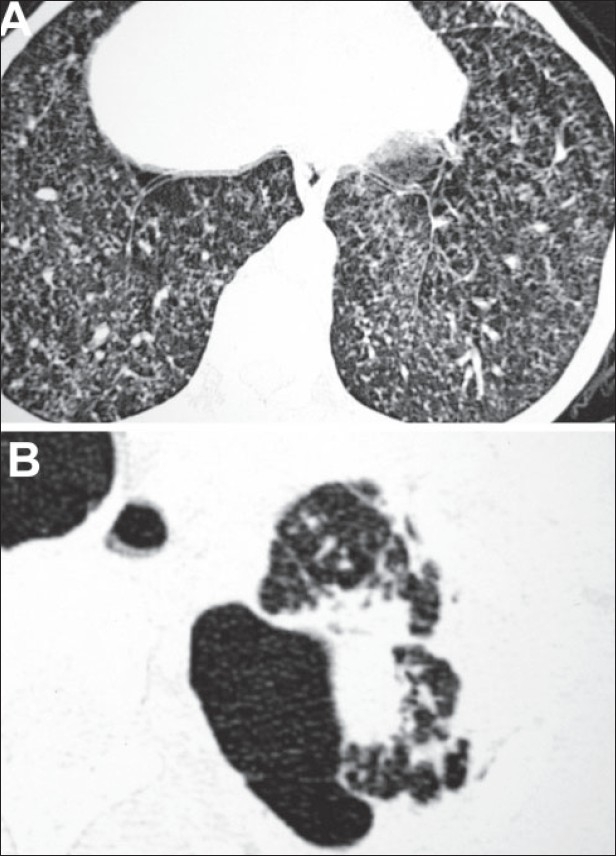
A. Computed tomogram revealing miliary opacities over bilateral lung fields. B. Computed tomogram showing multiple blebs over the left apex.
